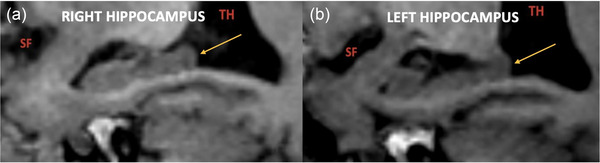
MR image of the right and left hippocampus in a patient with Parkinson's disease (PD): (a) right and (b) left hippocampus (yellow arrow) in the sagittal Fast Spoiled Gradient Recalled Echo (FSPGR) sequence. The left hippocampus volume is less than the right. In both images, enlargement of the lateral ventricular temporal horn (TH) and sylvian fissure (SF) is evident due to hippocampal atrophy.
